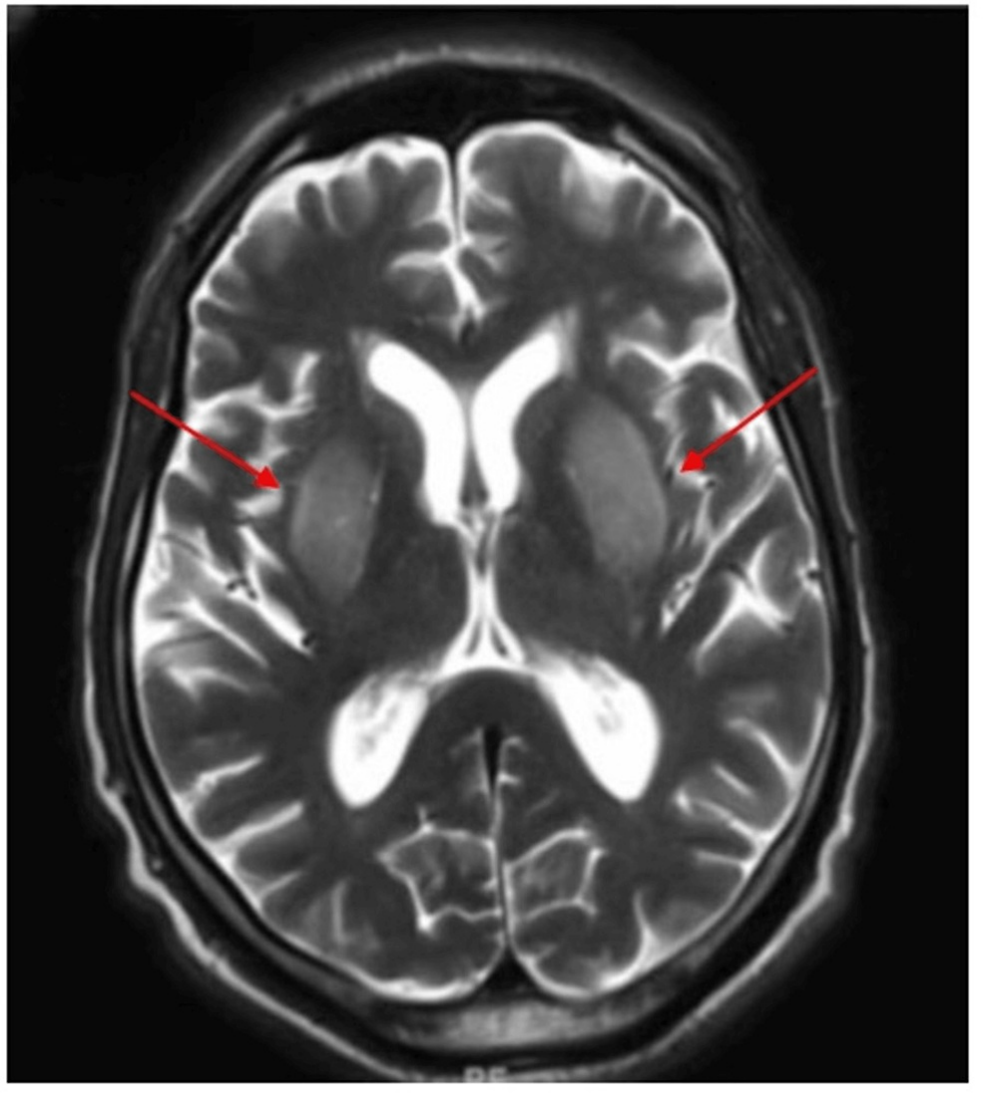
MRI brain: Day-one axial T2-weighted image shows symmetric hyperintense lesions in bilateral basal ganglia (red arrows)
